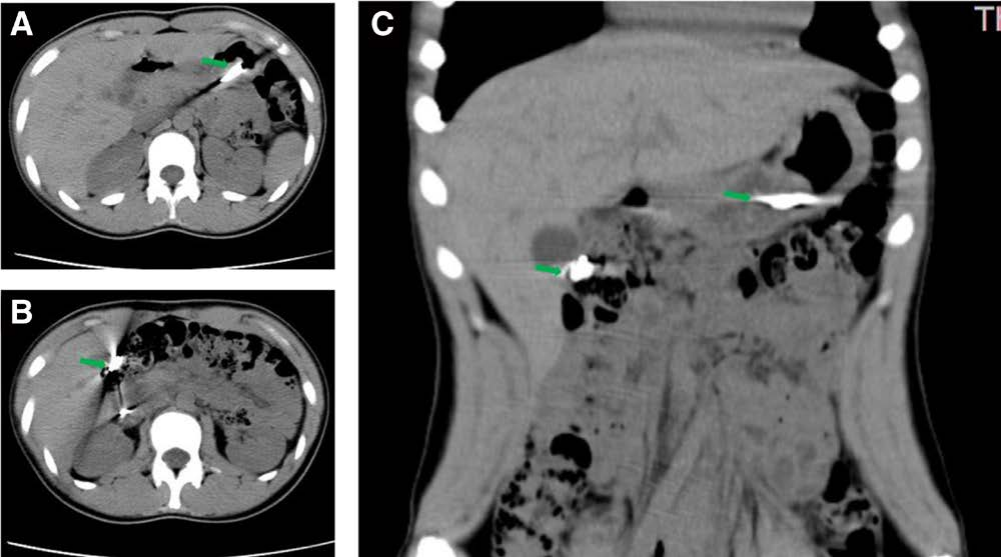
Abdominal CT showing dense shadows in the stomach and duodenum (A–C, green arrow). CT = computerized tomography.
